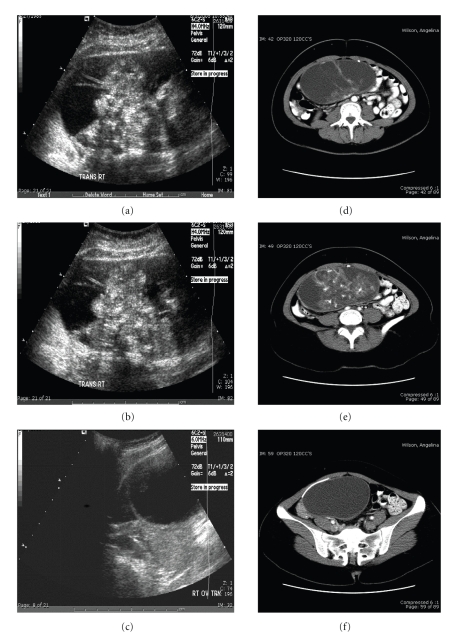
In figures (1a-1c), an abdominal ultrasound shows a large, walled, and multiseptated fluid collection. A small portion of the shunt catheter appears to be within this fluid collection, and thus the lesion was felt to be consistent with a pseudocyst, or CSFoma. In figures (1d-1f), a CT with IV contrast shows the same complex, multiseptated 16 × 8 × 17 cm pelvic mass emanating from the right ovary, with the distal shunt tubing draped circumferentially around the lesion, indicating that the shunt was not responsible. Surgical exploration leads to the diagnosis of teratoma.
